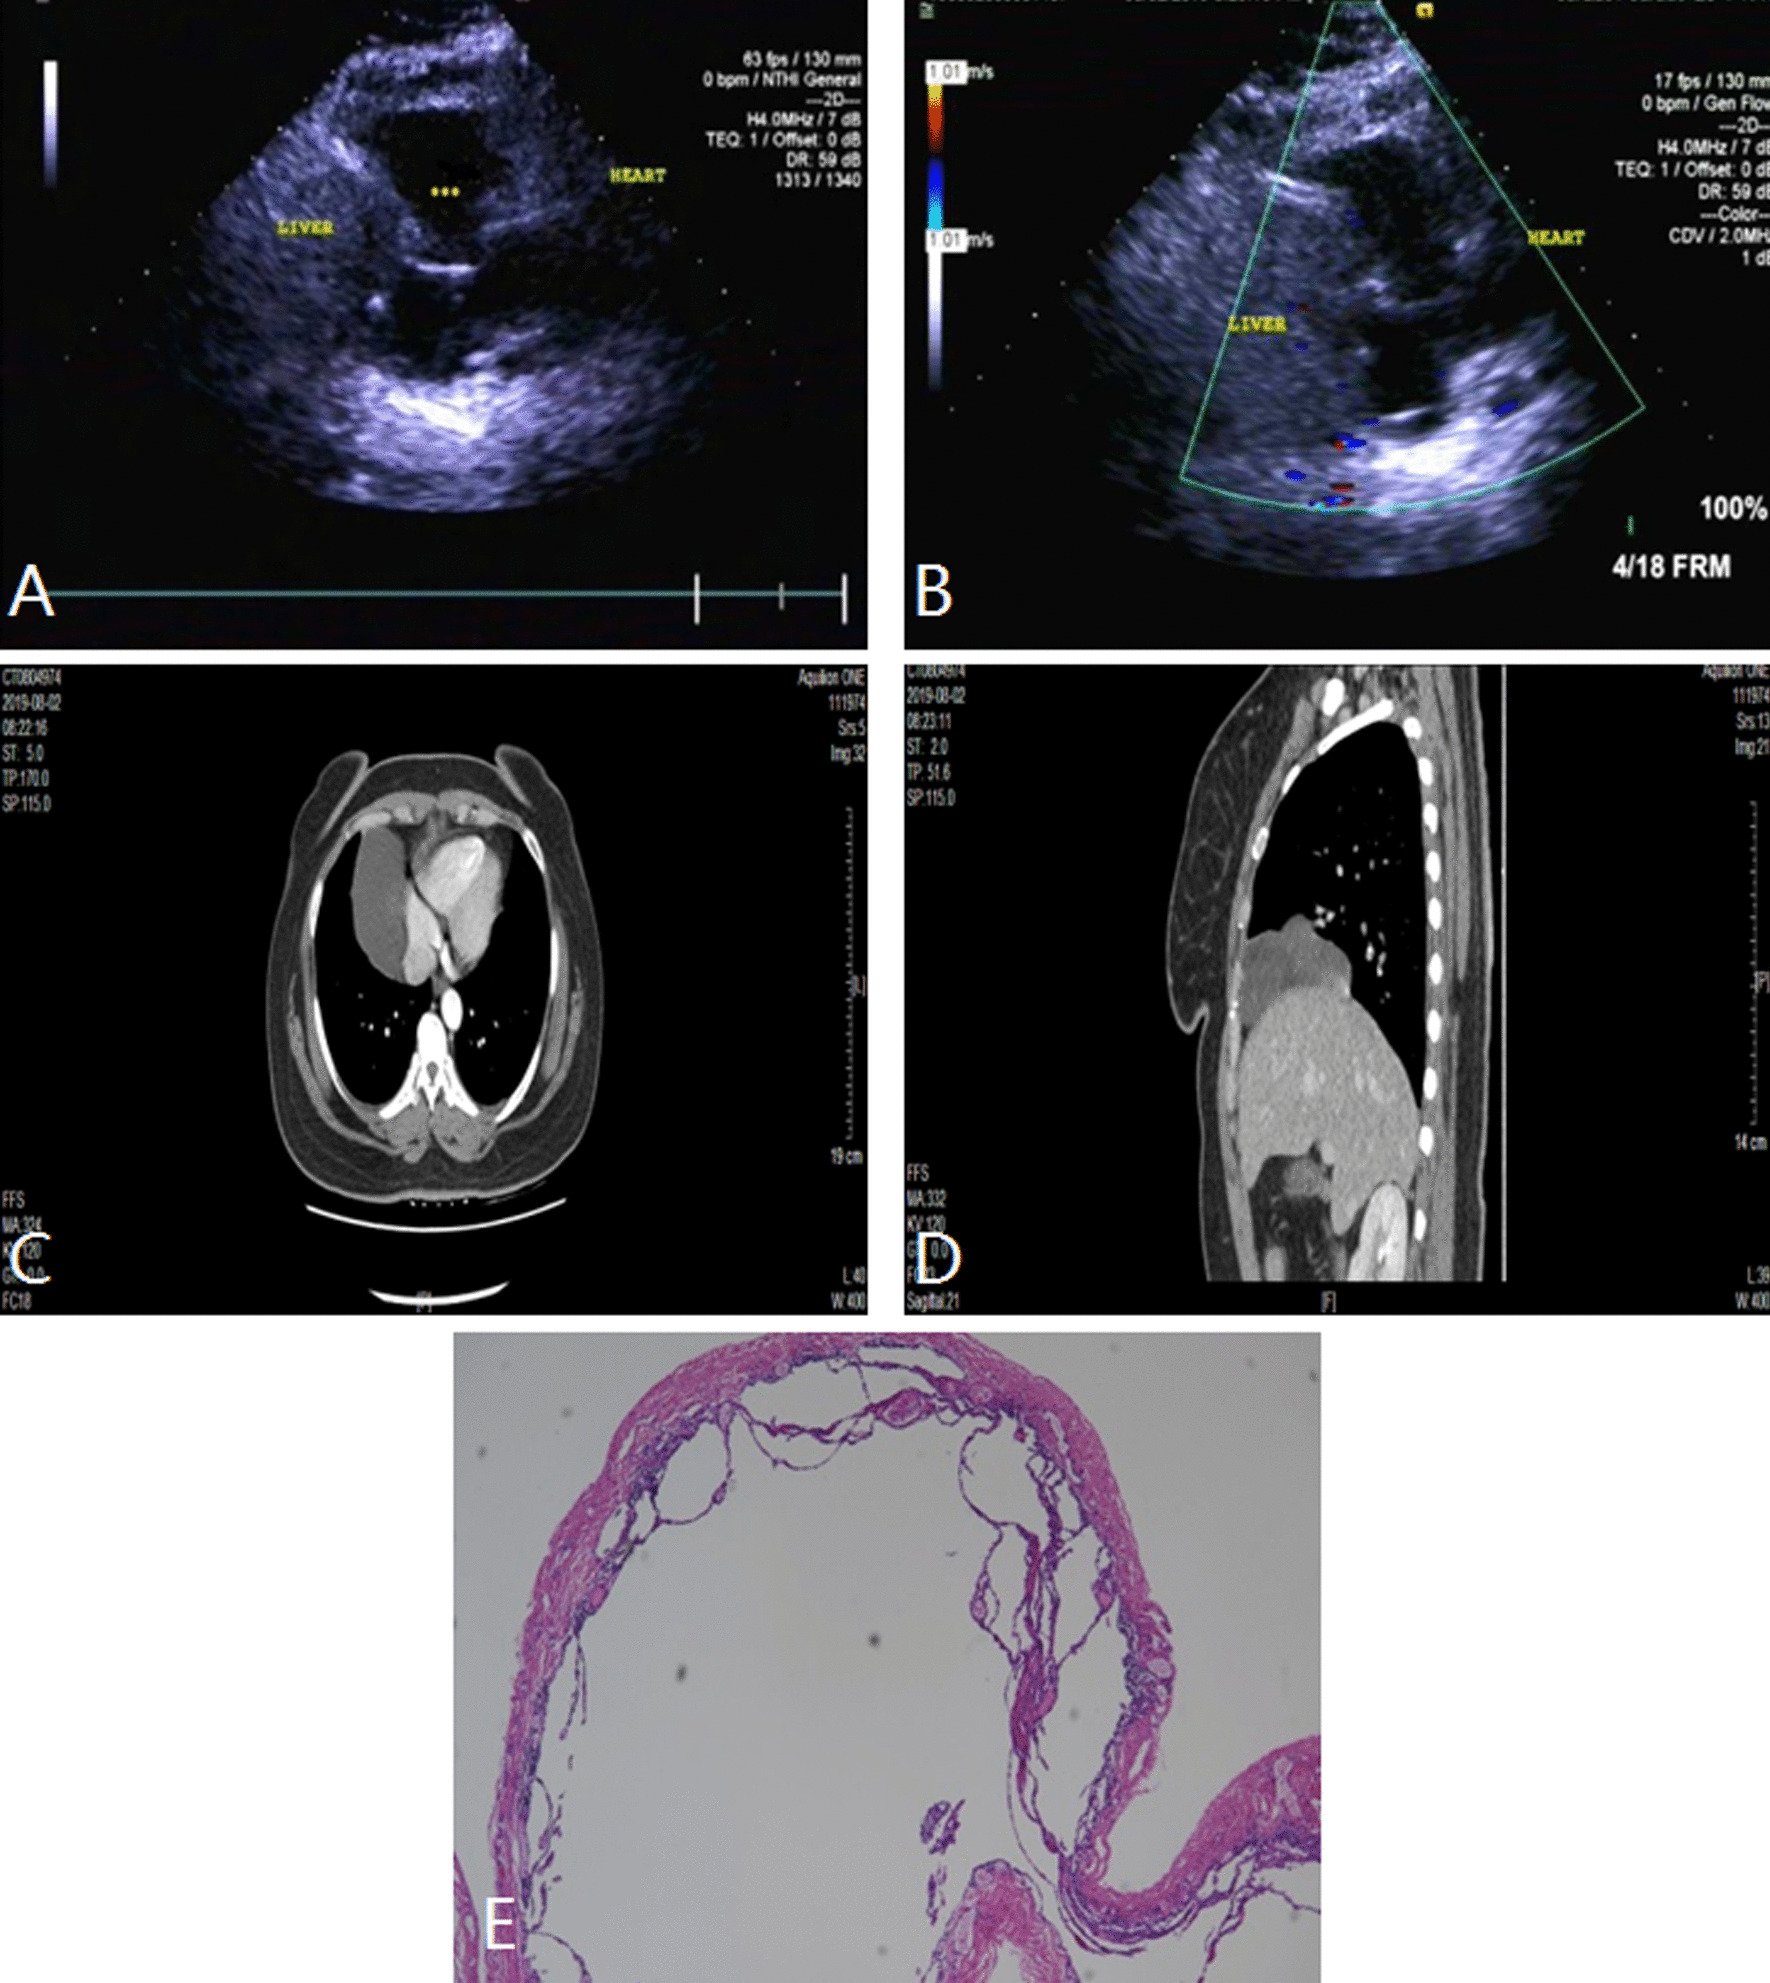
Middle mediastinal mass (pericardial cyst) in a 41-year-old woman. A and B TTE showed a well-circumscribed anechoic mass between the heart and the liver. C and D Contrast-enhanced CT showed a regularly shaped mass with the cystic hypodensity and no enhancement. E The pathologic diagnosis of this mass was pericardial cyst. The wall of the cyst was lined with cuboidal epithelium, and the cavity was filled with clear cystic fluid. TTE indicates transthoracic echocardiography; CT, computer tomograph; asterisks, mass
